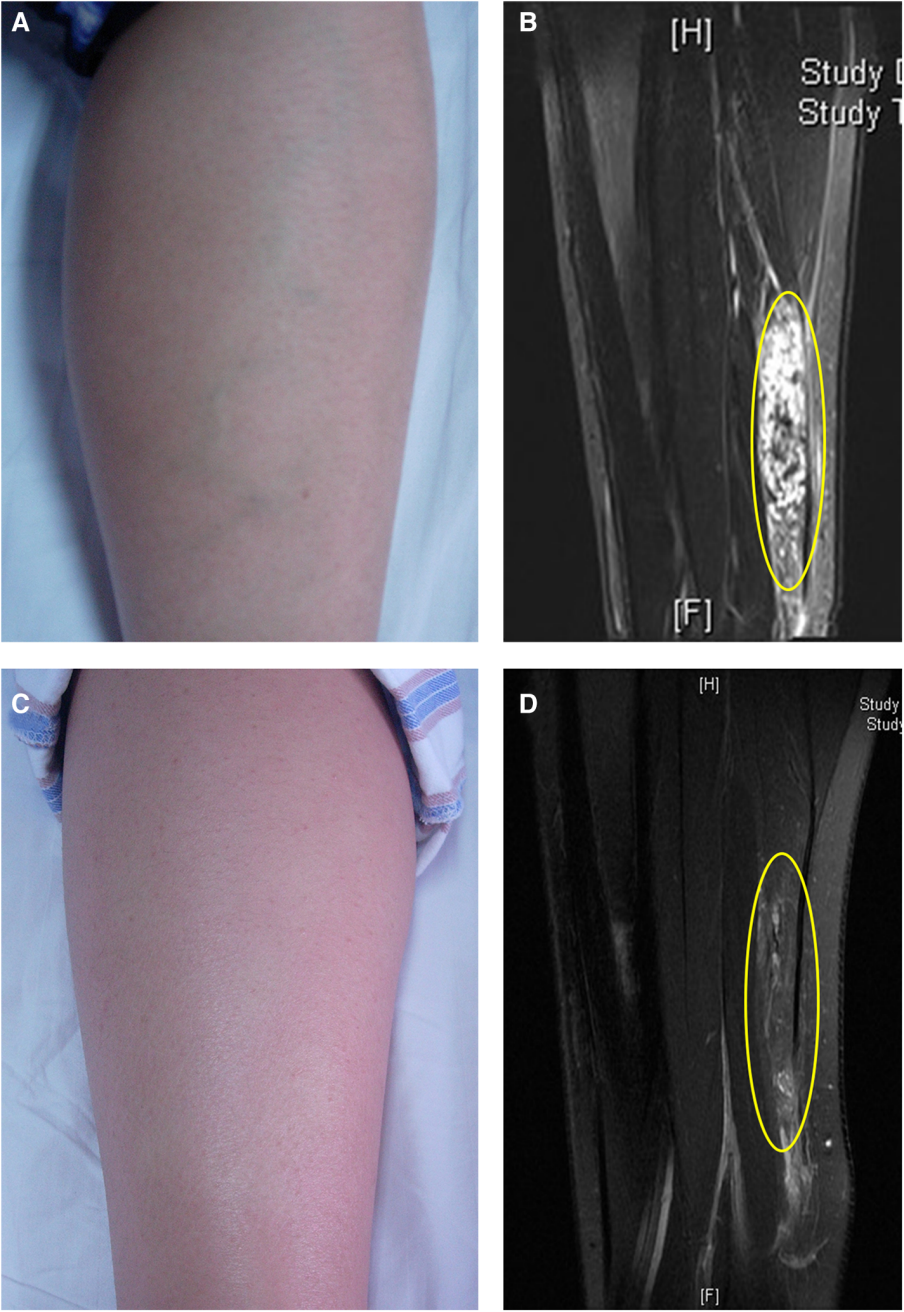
Focal mass type IMVM in the left calf. (A) The appearance before treatment. (B) The MRI before treatment. The yellow ellipse indicated that the lesion was located in the gastrocnemius muscle. (C) The appearance after three times of treatment. (D) The MRI after treatment. The yellow ellipse indicated the lesion. The copyrights of the figures were held by corresponding author.
